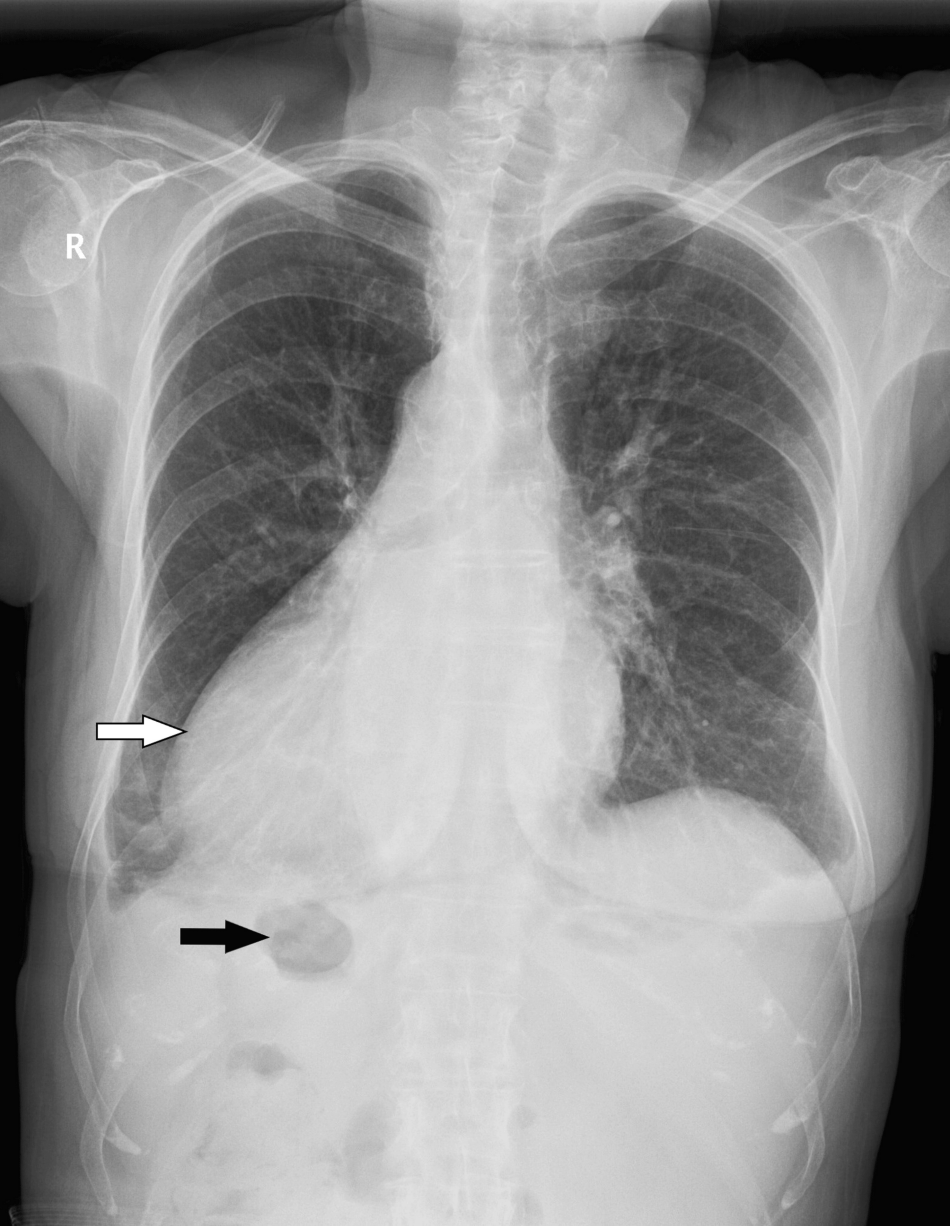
X-ray of the heart and lungs showing the upper part of the abdomen. Dextrocardia (white arrow) and the stomach (black arrow) are visualized on the right side. There are no signs of pneumoperitoneum.
